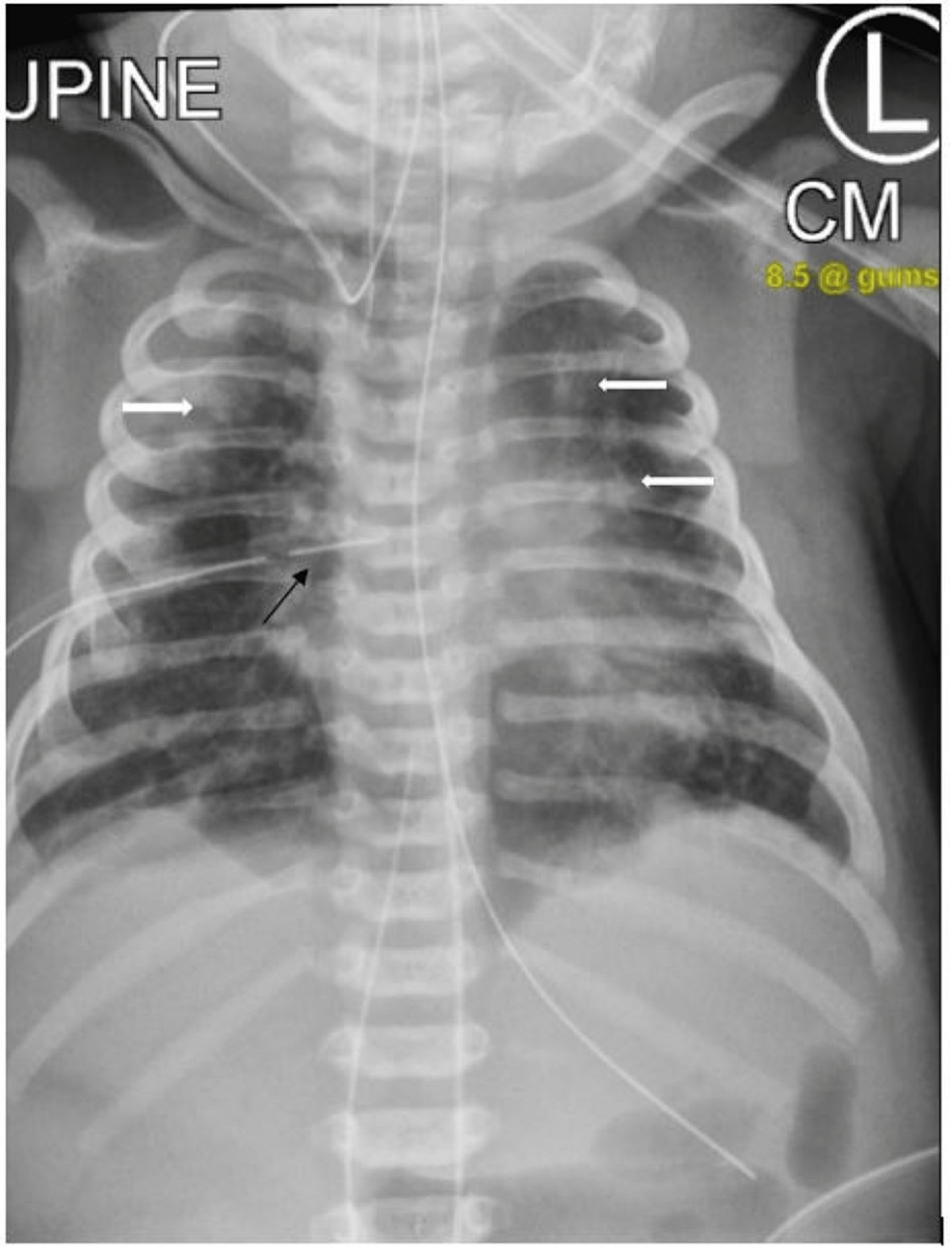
Chest X-ray, consistent with meconium aspiration syndrome (White arrows), shows resolved right tension pneumothorax, appropriate position of endotracheal tube, orogastric tube, umbilical lines, and right chest tube (Black arrow)
